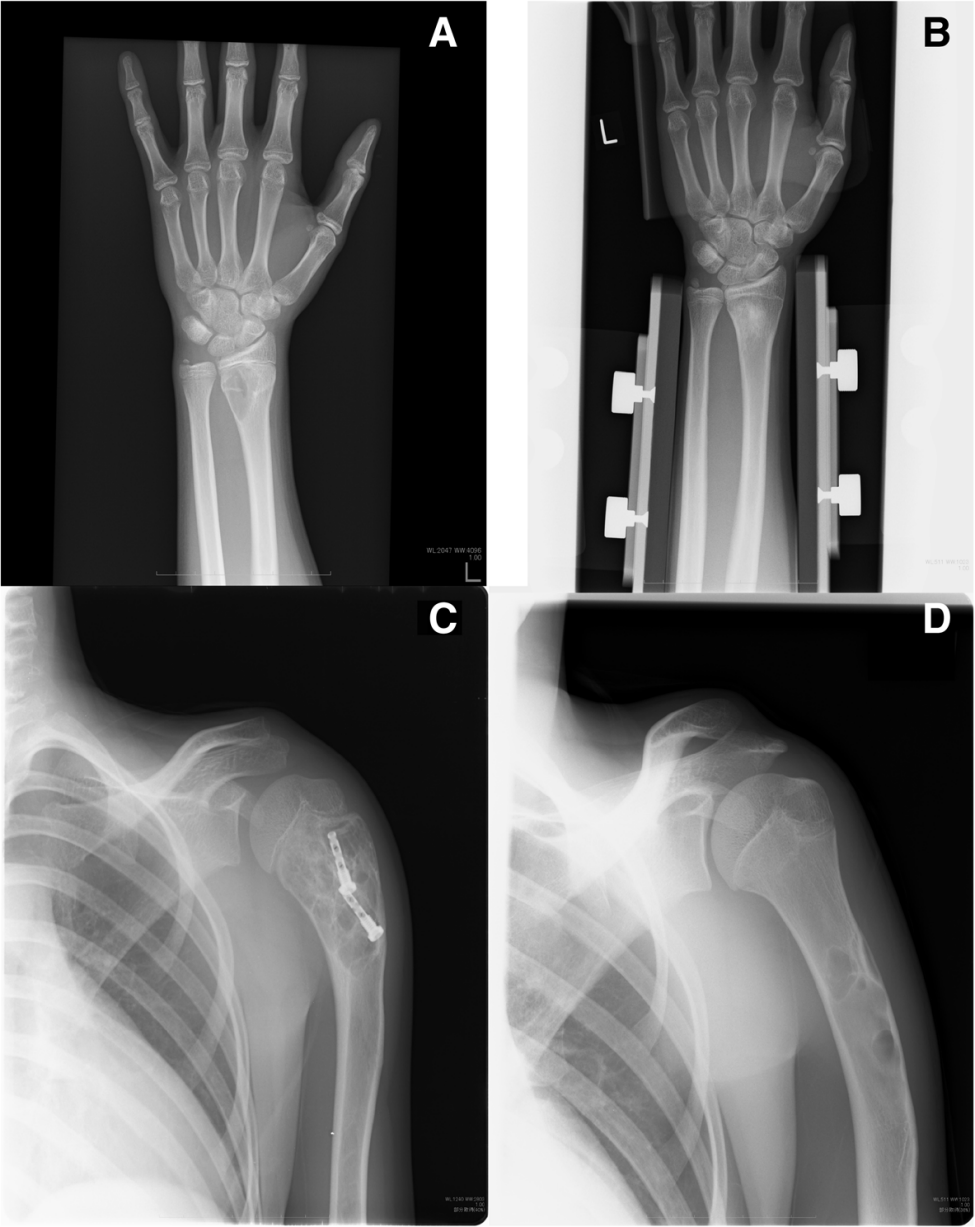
Healing of UBC. Fifteen-year-old male of UBC in his left distal radius: Anteroposterior radiograph at his first presentation (A) and 12 months later (B). He had a pathological fracture at first visit and complete healing was observed after observation. Ten-year-old male of UBC in his left proximal humerus: Anteroposterior radiograph after surgery (C) and 4 years 7 months after surgery (D). Partial healing was observed after surgery of cannulated screw.
